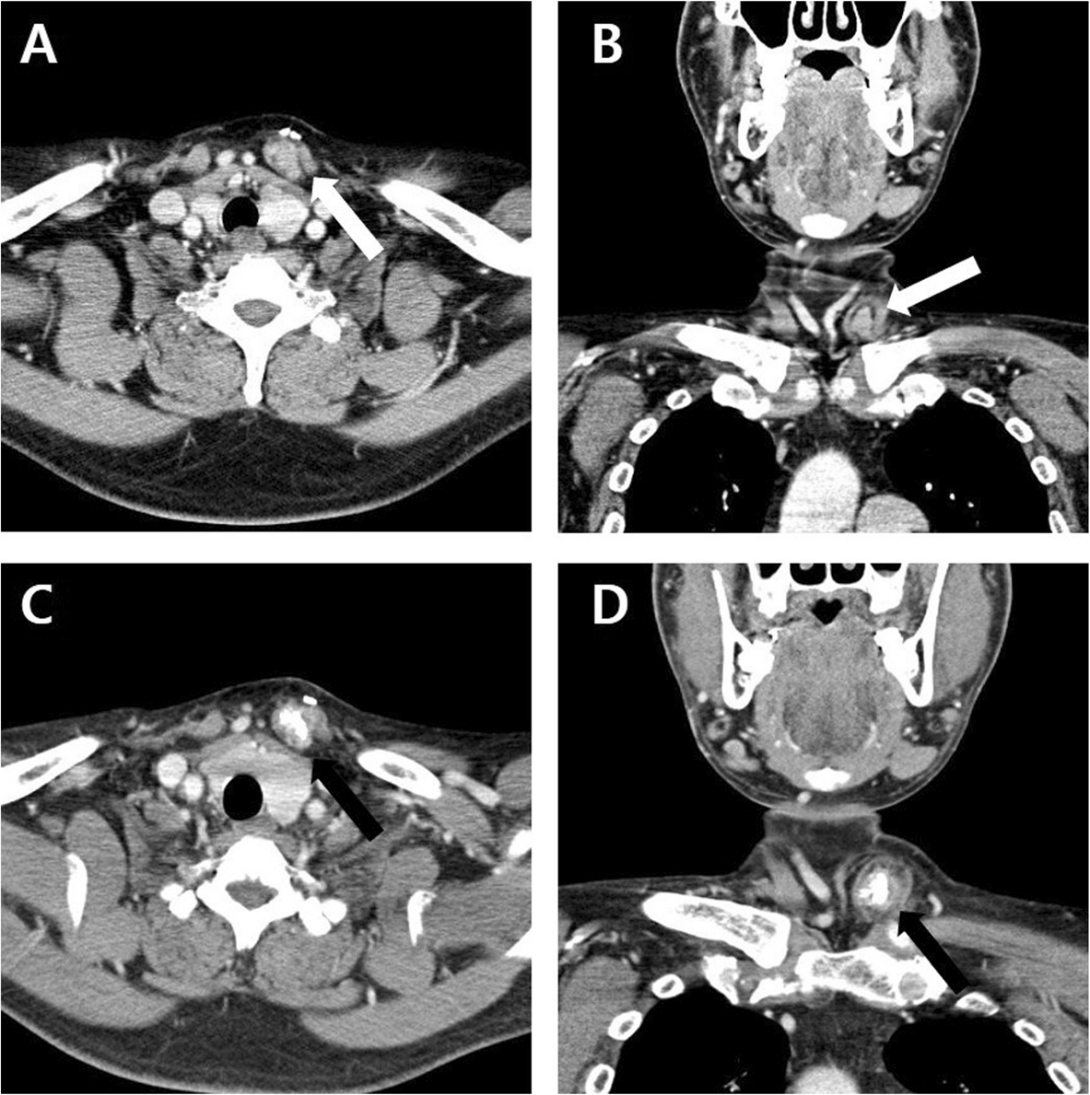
Postoperative follow up neck CT. a b An enlarged mass considered as transplanted parathyroid tissue (2.0 × 1.7 × 1.7 cm in size) in the clavicular head of left sternocleidomastoid muscle (white arrow). Enhanced Neck CT performed 3 years after total parathyroidectomy with auto-transplantation. (a) Axial view; (b) Coronal view; (c)(d) Compared with 3 years after surgery, a more enlarged mass with internal dense calcification (2.5 × 2.0 × 2.0 cm in size) was found on the clavicular head of left sternocleidomastoid muscle (black arrow). Enhanced Neck CT performed 8 years after total parathyroidectomy with auto-transplantation. (c) Axial view; (d) Coronal view
